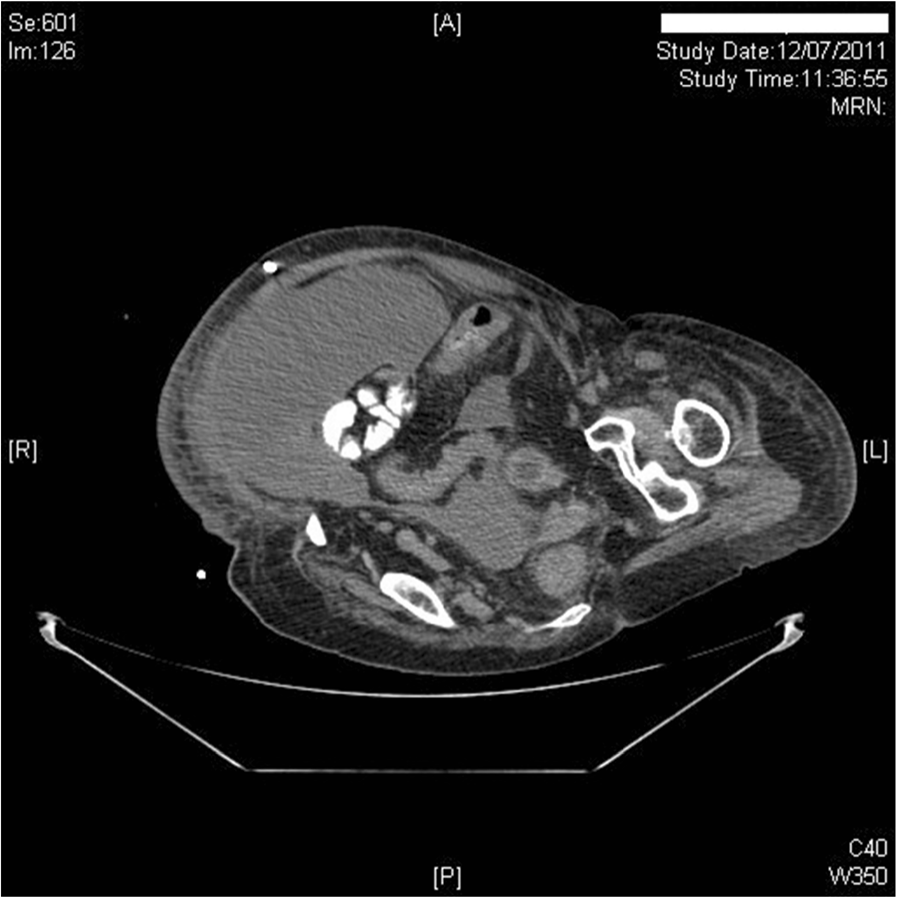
Computed Tomography of chest, abdomen and pelvis, performed after percutaneous drainage of right pyonephrosis: a large fluid collection was seen in the abdomen extending into the pelvis with enhancing walls. Fluid was also noted around the spleen, in the paracolic gutters and in the root of the mesentery.
